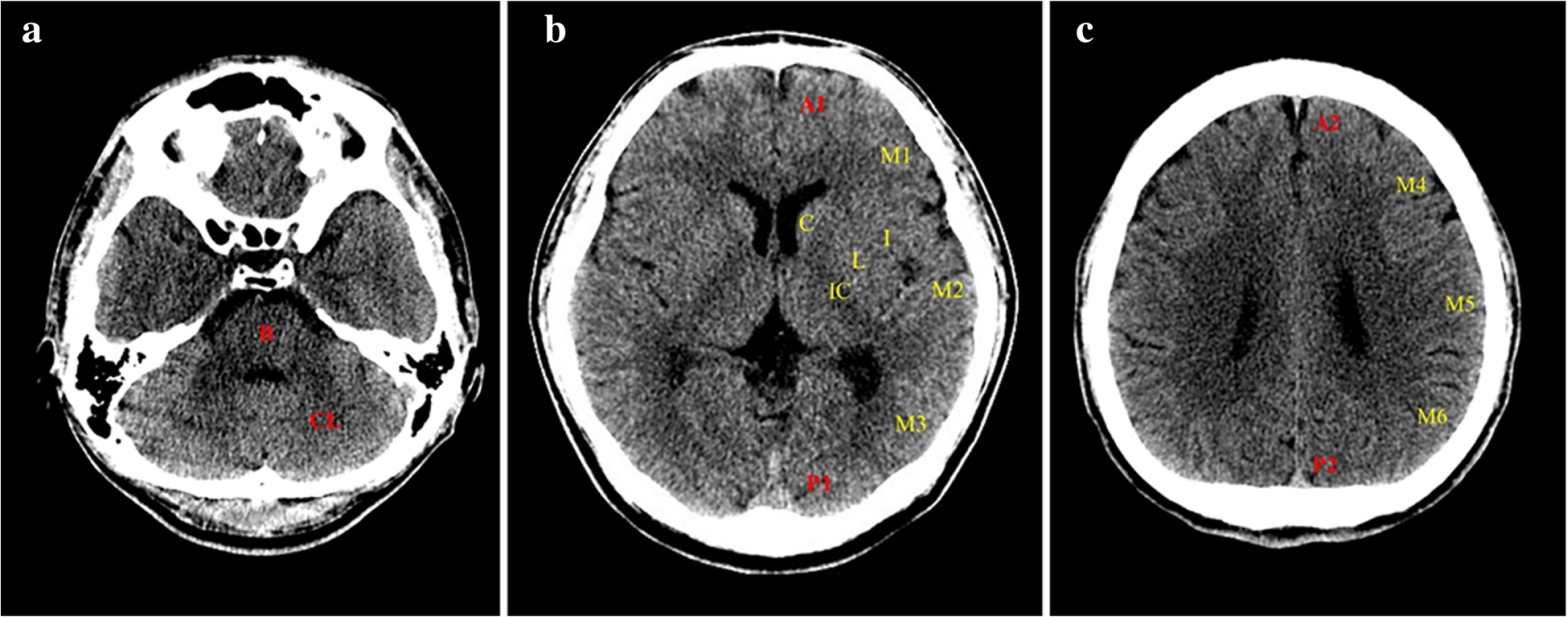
Axial computed tomographic images at three levels (a, b, c). Modified Alberta Stroke Program Early Computed Tomography Score (ASPECTS) was used for analysis of images. The score was used to interpret the whole brain. It was not limited to specific slices. *See* Table 1 for further details. Bilateral yellow regions indicate ASPECTS-b (extended to both sides from the original ASPECTS protocol). Combined bilateral regions of yellow and red indicate modified ASPECTS. *A1* Anterior cerebral artery (ACA) lower, *A2* ACA upper, *ASPECTS-b* Bilateral ASPECTS, *B* Brainstem, *C* Caudate, *CL* Cerebellum, *I* Insula, *IC* Internal capsule, *L* Lentiform nucleus, *M1–M6* Middle cerebral artery territories, *mASPECTS* Modified ASPECTS, *P1* Posterior cerebral artery (PCA) lower, *P2* PCA upper
